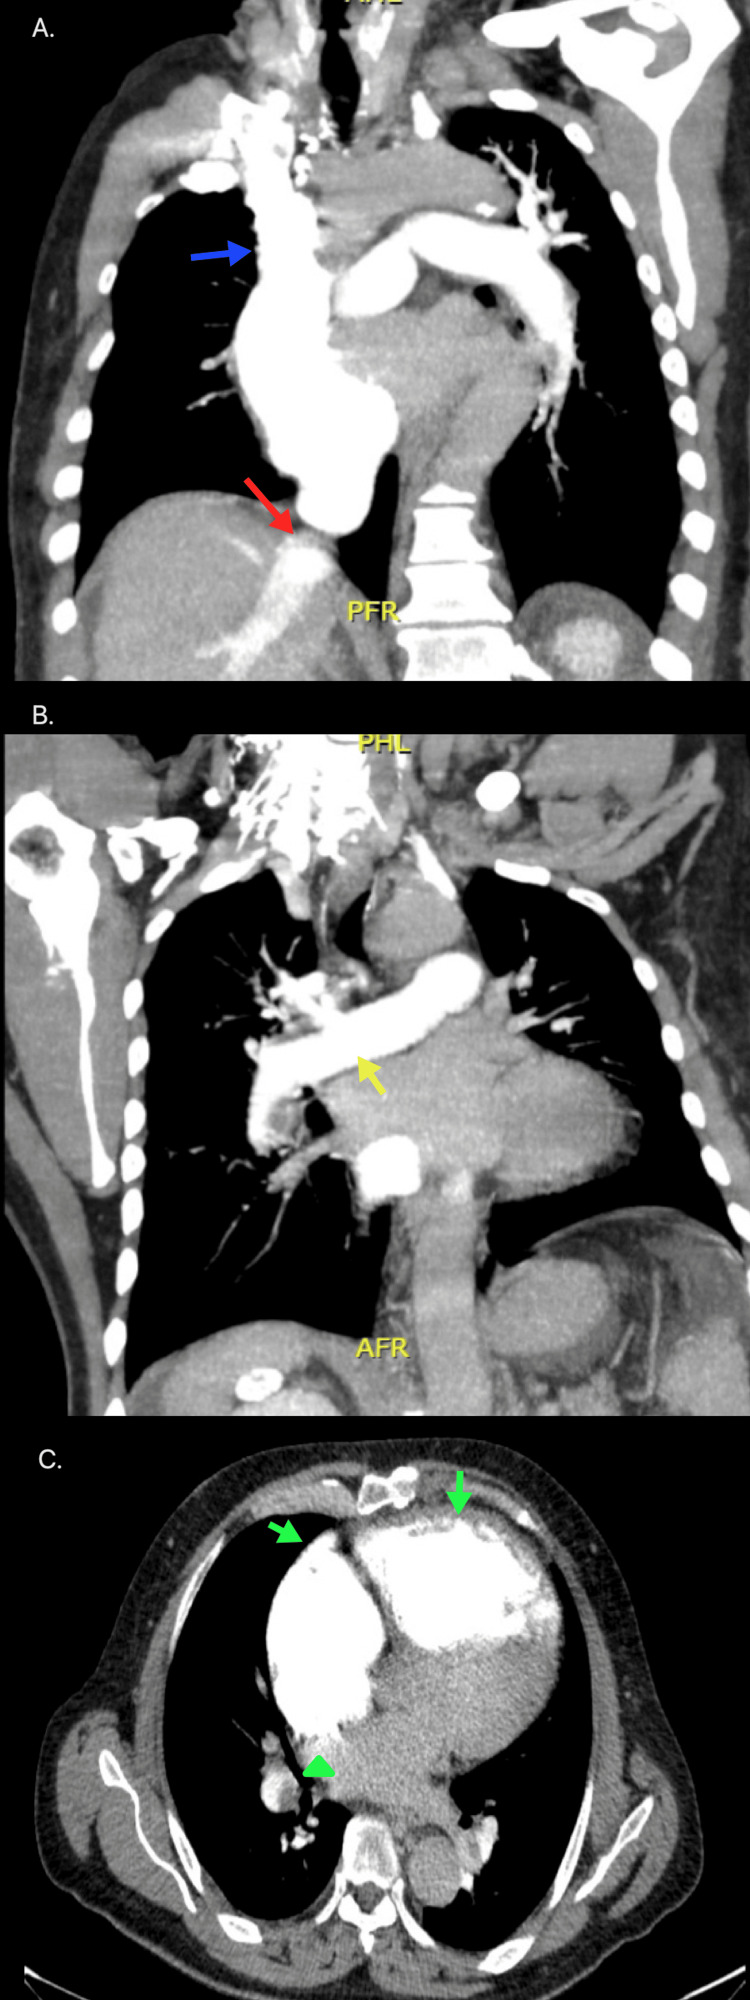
Pulmonary embolism A 60-year-old male patient with severe pulmonary hypertension and JAK2-positive PV. The following findings were observed in chest CT angiography: (A) Dilatation of the superior vena cava (blue arrow) with contrast reflux into the inferior vena cava and opacification of the hepatic veins, indicative of right-sided heart failure (red arrow). (B) Dilation of the pulmonary artery (yellow arrow). (C) Prominence of right-sided cavities (green arrow), with eccentric filling defects involving lobar and segmental arteries, suggestive of chronic pulmonary embolism (green arrowhead). PV, polycythemia vera Image courtesy: Pulmonology Service of the Internal Medicine Department, University of Antioquia. Consent for open access publication was obtained from the patient.
